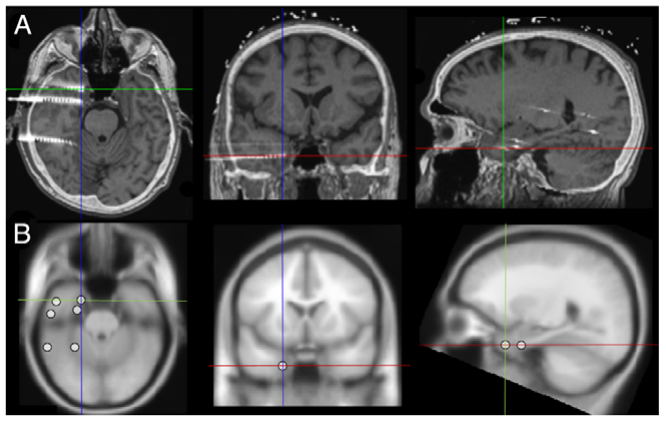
Electrodes contacts and corresponding ROI locations. (a) Image fusion between CT-scan obtained during implantation of electrodes and 3D T1 volume acquired after electrode implantation. (b) Definition of ROIs corresponding to the selected contacts.
